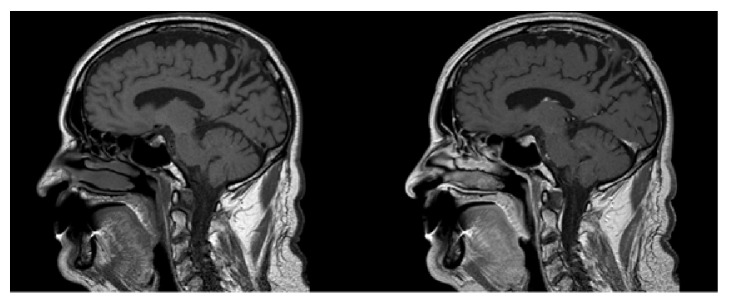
Sagittal T1-weighted images without and with contrast show brain herniation to the bone defect, without enhancement.
